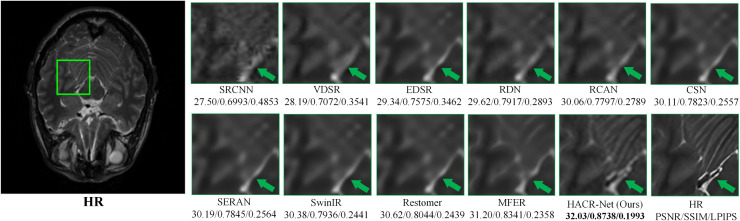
Visual comparison of different SR methods on the T2-weighted IXI dataset at × 4 magnification. The green box highlights the zoomed-in region, enabling evaluation of structural fidelity and reconstruction detail.
